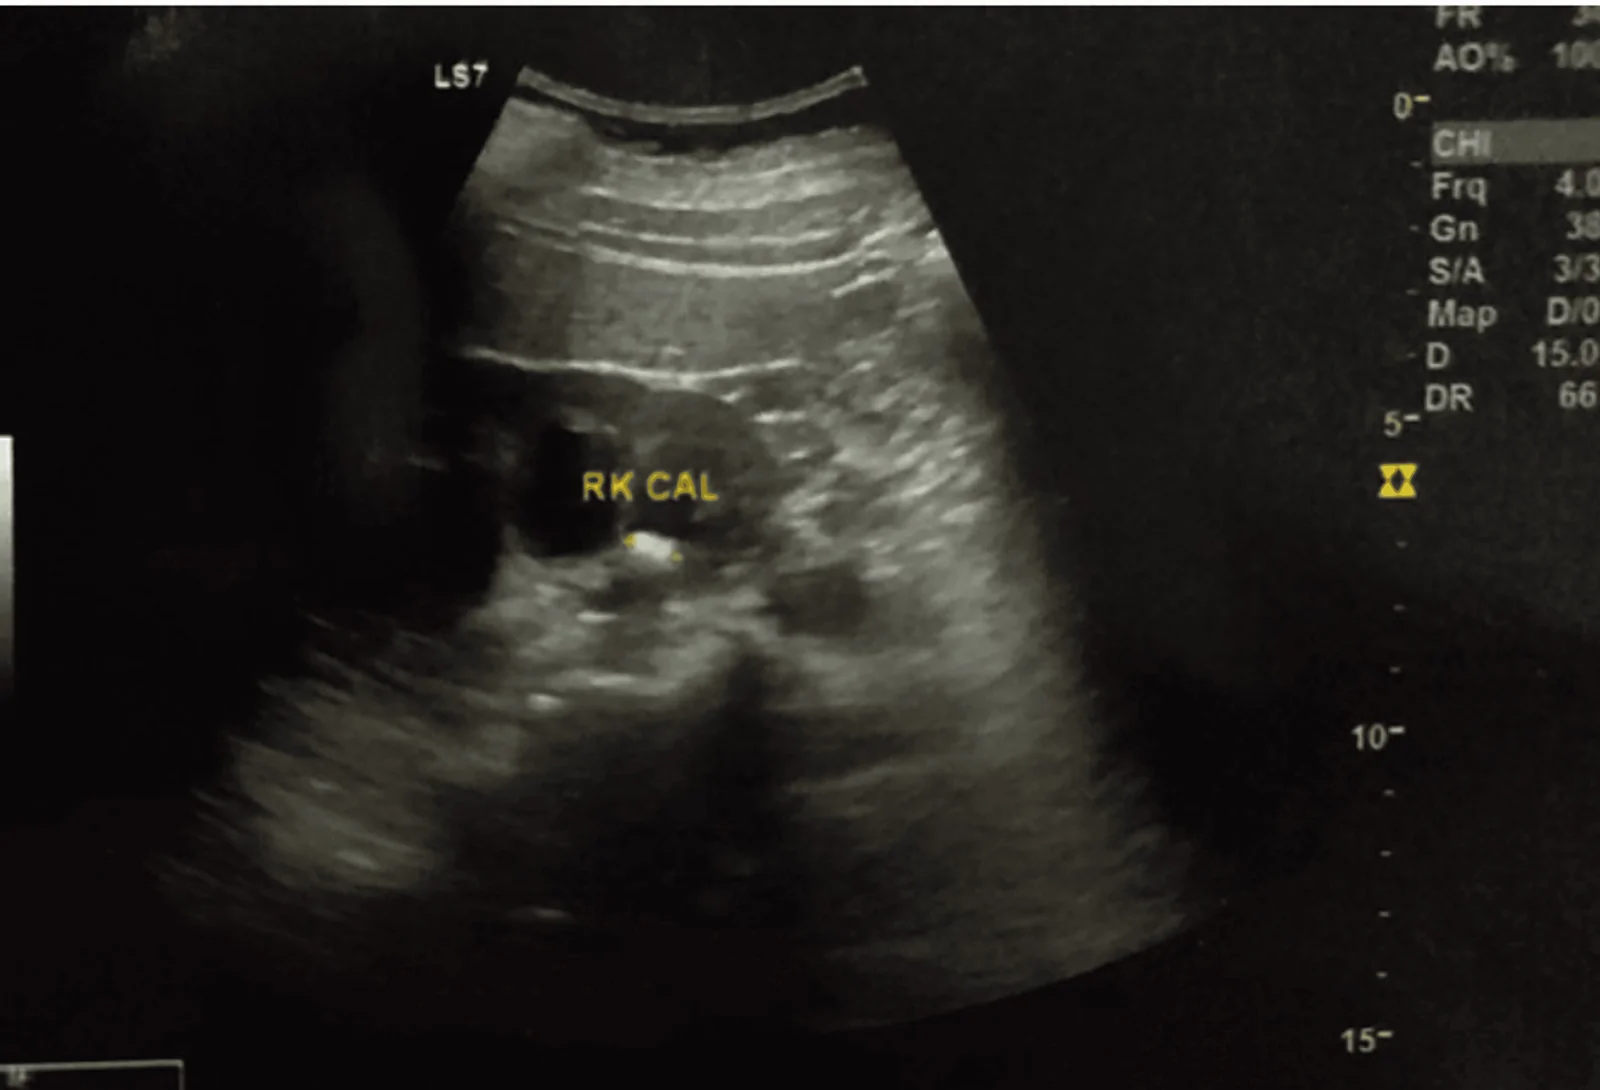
Ultrasonography showing 3 cm right renal pelvic calculus.
